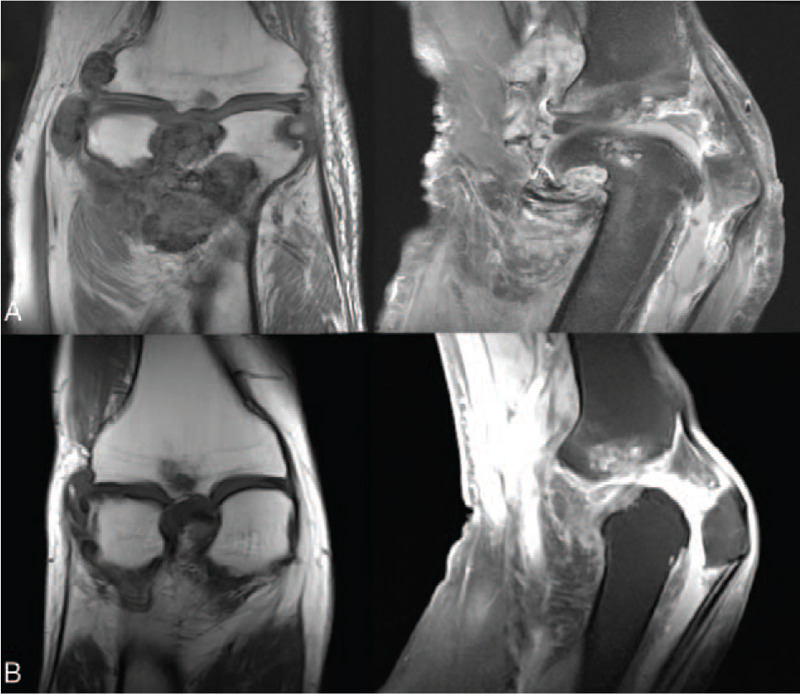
(A) Postoperative MR images demonstrate nodular and flocculent thickening of the right knee joint's synovium and the focus involved and invaded the surrounding tissue (patient 1). (B) Postoperative MR image showed that the left knee joint's synovium was uneven, widely thickened, and multiple nodular abnormal signals could be seen. (patient 2).
